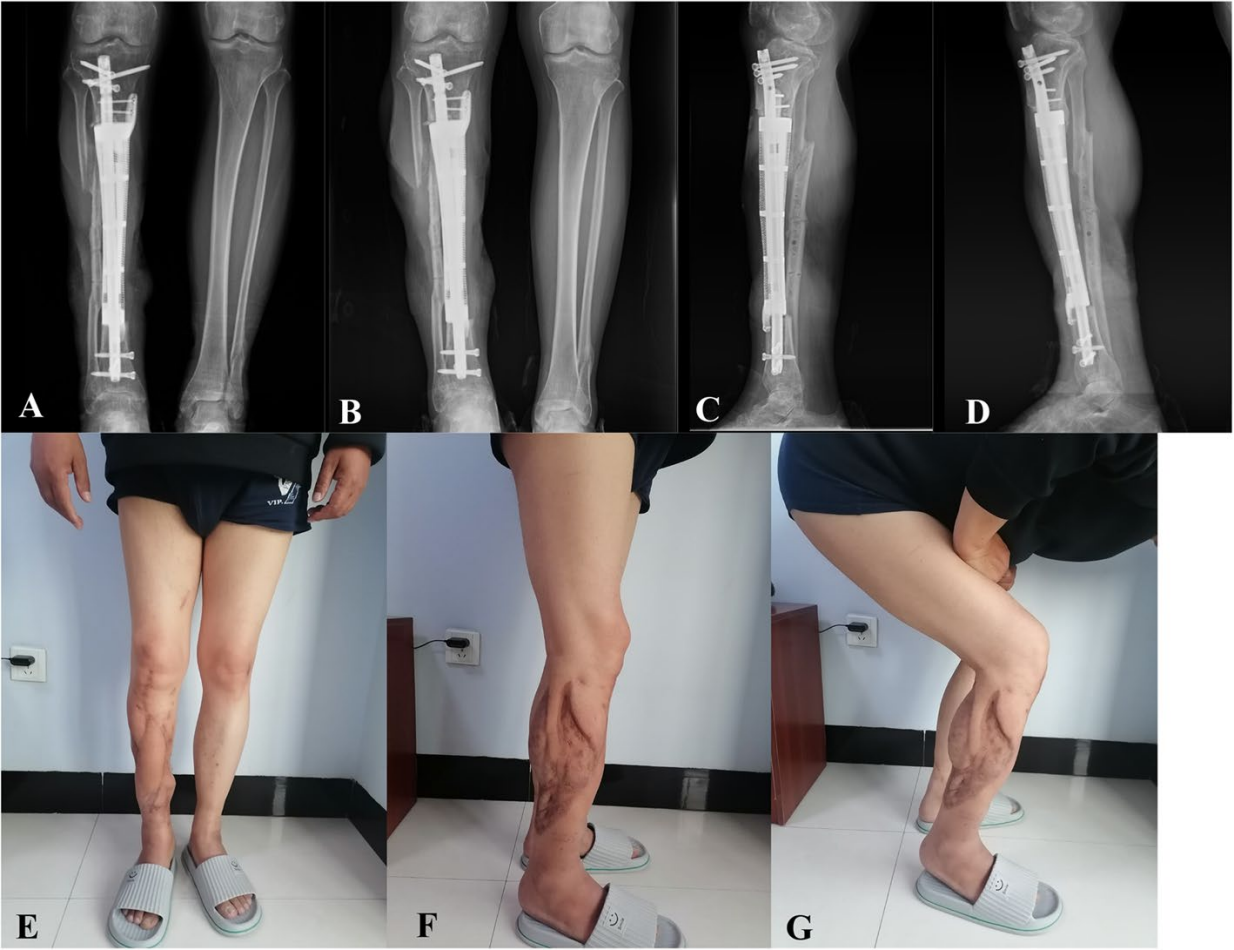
A, B Anteroposterior radiographs of the immediate postoperative (A) and 12-month follow up (B), showing the stability of the reconstruction. C, D Lateral radiographs of the immediate postoperative (C) and 12-month follow up (D). Continuous callus formation across the bone interface could be observed on the proximal. E,F,G The wound heal well with satisfactory appearance and could satisfy the needs of daily social work
